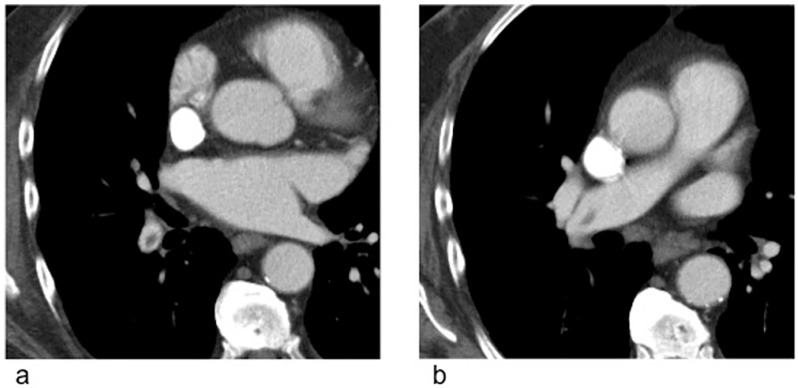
Follow up examination of patient Nr. 4 (see Table 2) 12 months later. While the CT evaluated in this study showed only a small subsegmental peripheral embolus (Fig 3c), the follow up CT demonstrated multiple lobar and central pulmonary emboli.
